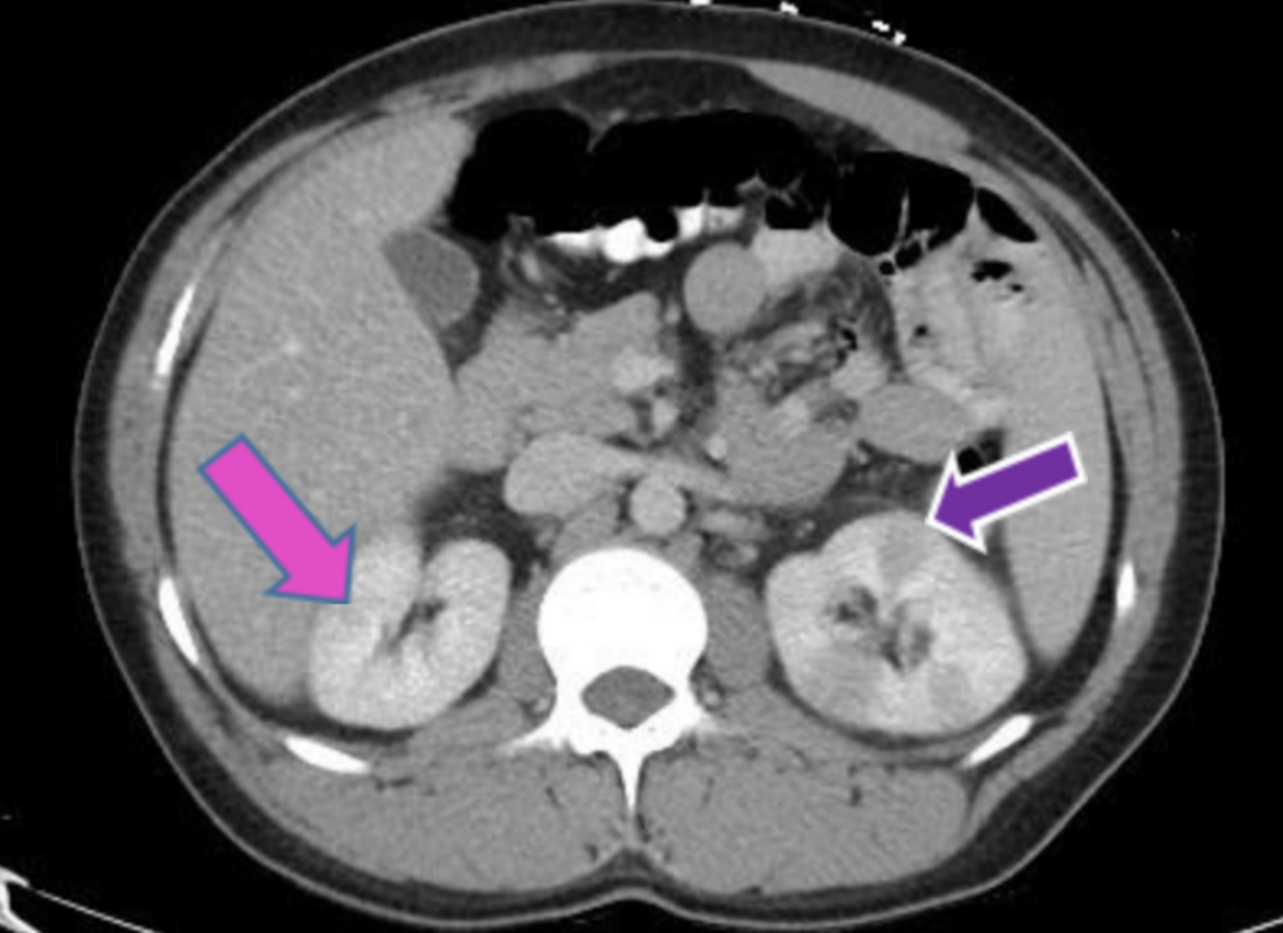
CT scan showing darkening of kidneys shows pyelonephritis indicated by the pink and purple arrows
